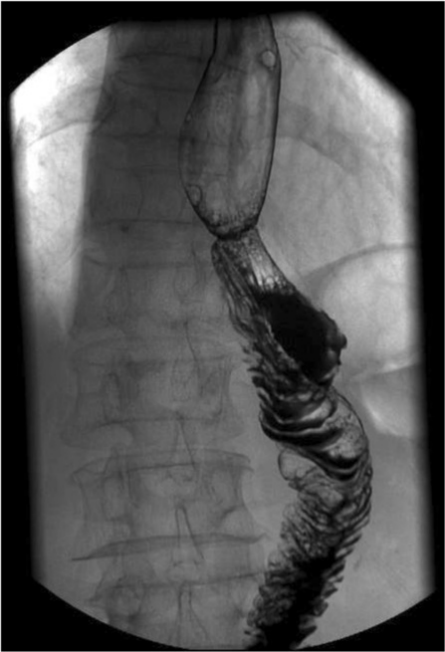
Upper gastrointestinal contrast X-ray check of esophagojejunostomy at postoperative 1 month.
